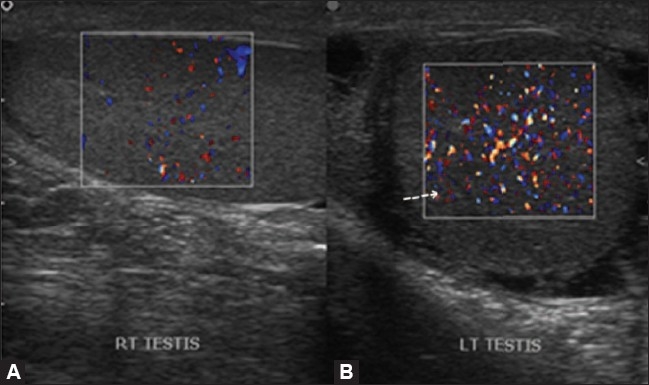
Orchitis. Color Doppler shows increased flow in the left testis (arrow in A) with normal color flow in the right testis
